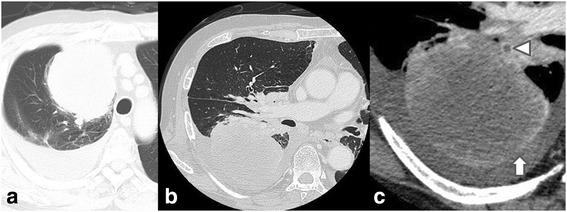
a Plain CT at first visit showing a large tumor mainly located at right S1-3, widely in contact with the precordial mediastinum and pleura. Massive pleural effusion in the right thoracic cavity is also present. Contrast-enhanced CT image at the onset of torsion, b lung window, c mediastinal window (enlarged image). The tumor in the right upper lung lobe has moved to the posterior thoracic space. There is a thrombus in the upper pulmonary vein (*triangle*) and non-contrast regions in the peripheral tumor (*upward arrow*), leading to suspicion of blood flow impediment
